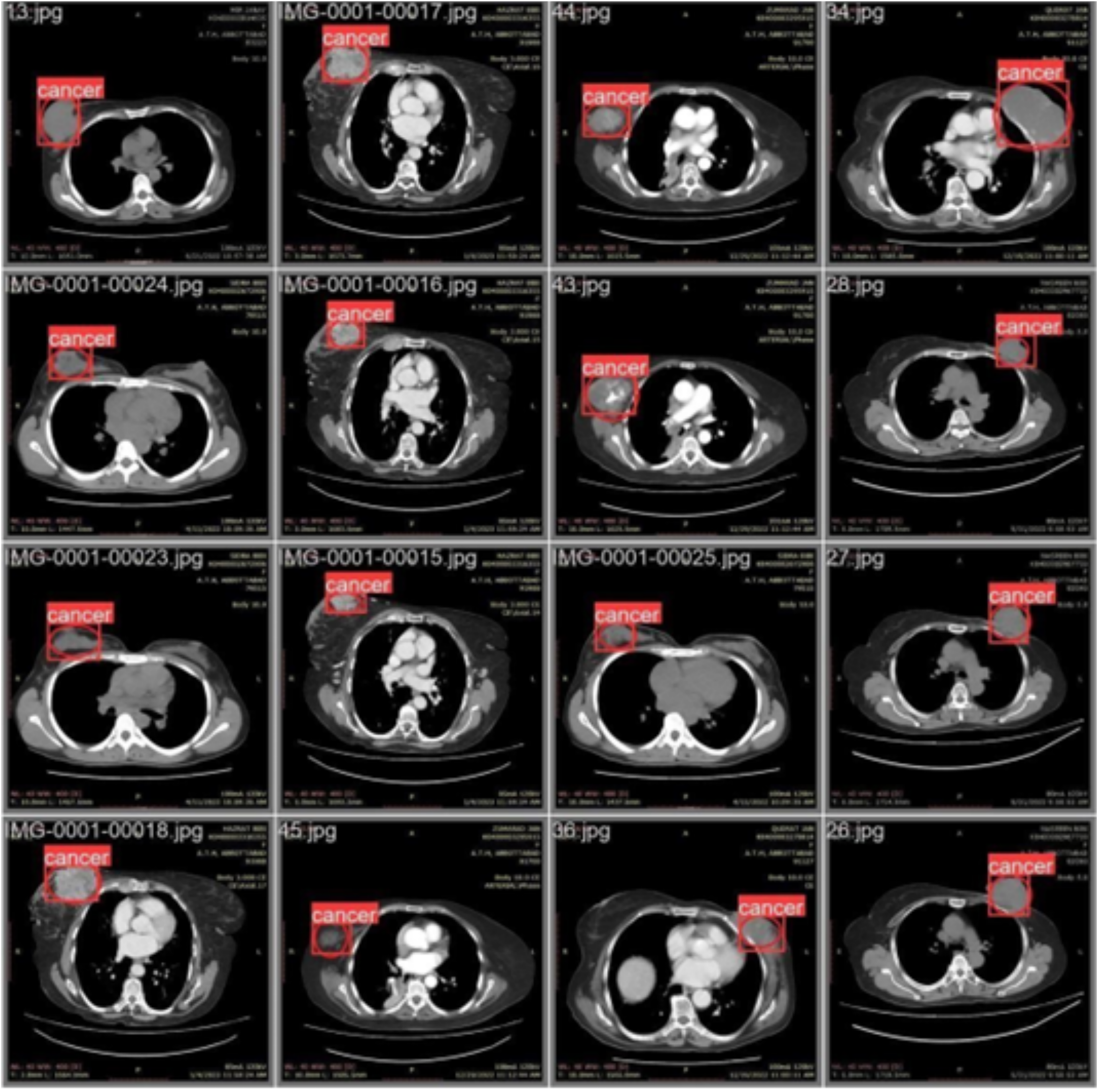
CT scan of breast cancer of 16 diseased patients with predicted regions of breast cancer during validation.
